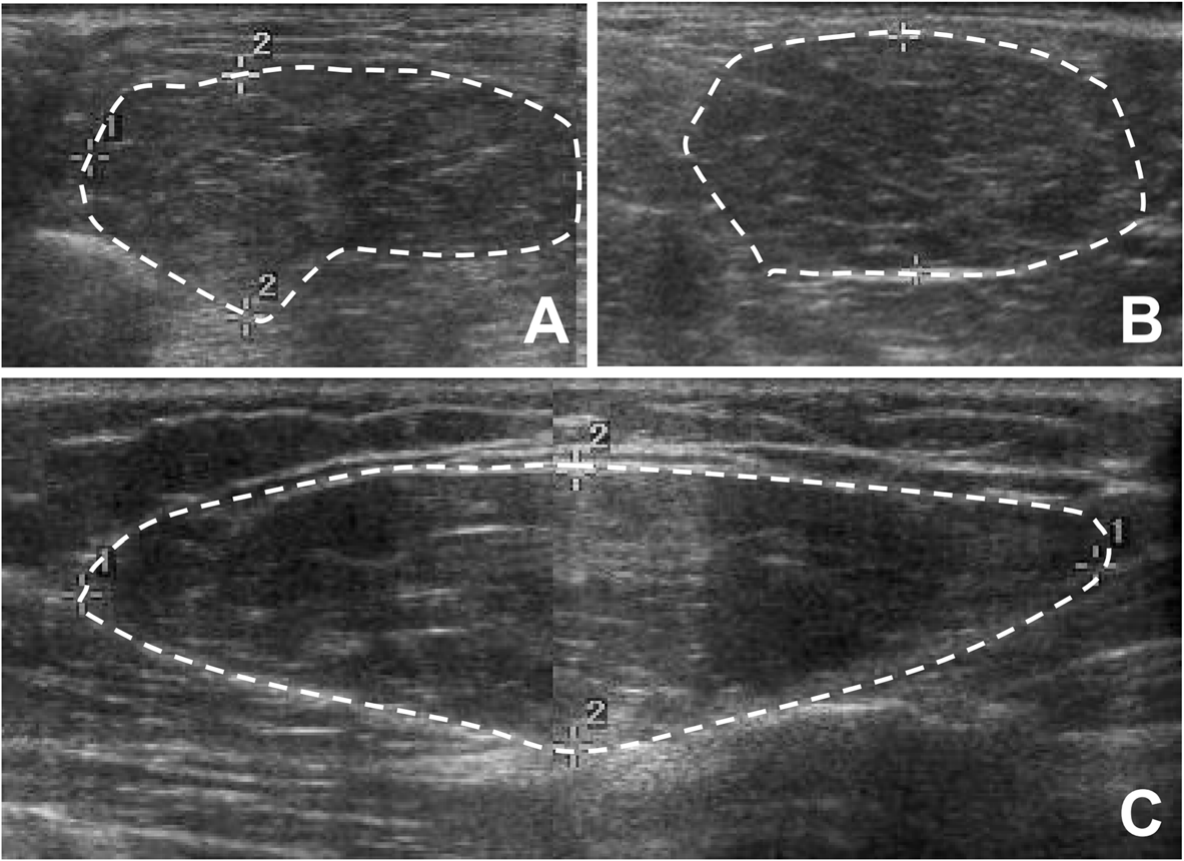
Ultrasonography findings. Ultrasonography indicated excessive adipose tissue (white lines) located in the subcutaneous layer of the shoulders (a, 5.73 × 2.12 × 4.32 cm), posterior neck (b, 4.7 × 7.7 × 2.0 cm), and back (c, 8.2 × 8.6 × 2.2 cm). All masses were compressible and had minimal vascularity
